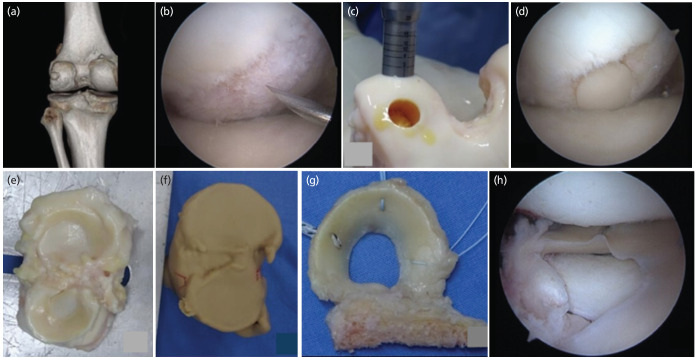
Intra-surgical findings and 3D model for surgical planning. Arthroscopic views and harvesting of osteochondral allografts. (a) Computed tomography of the left knee with 3D reconstruction. (b) Chondral lesion of the lateral femoral condyle in weight-bearing area. (c) Harvesting of osteochondral allografts. (d) Chondral lesion in the lateral femoral condyle covered by 80%. (e) Tibial plateau allograft. (f) Life-size 3D model of the proximal tibia. (g) Allograft preparation for meniscal transplantation using the dovetail technique. (h) Arthroscopic view of the transplanted lateral meniscus.
